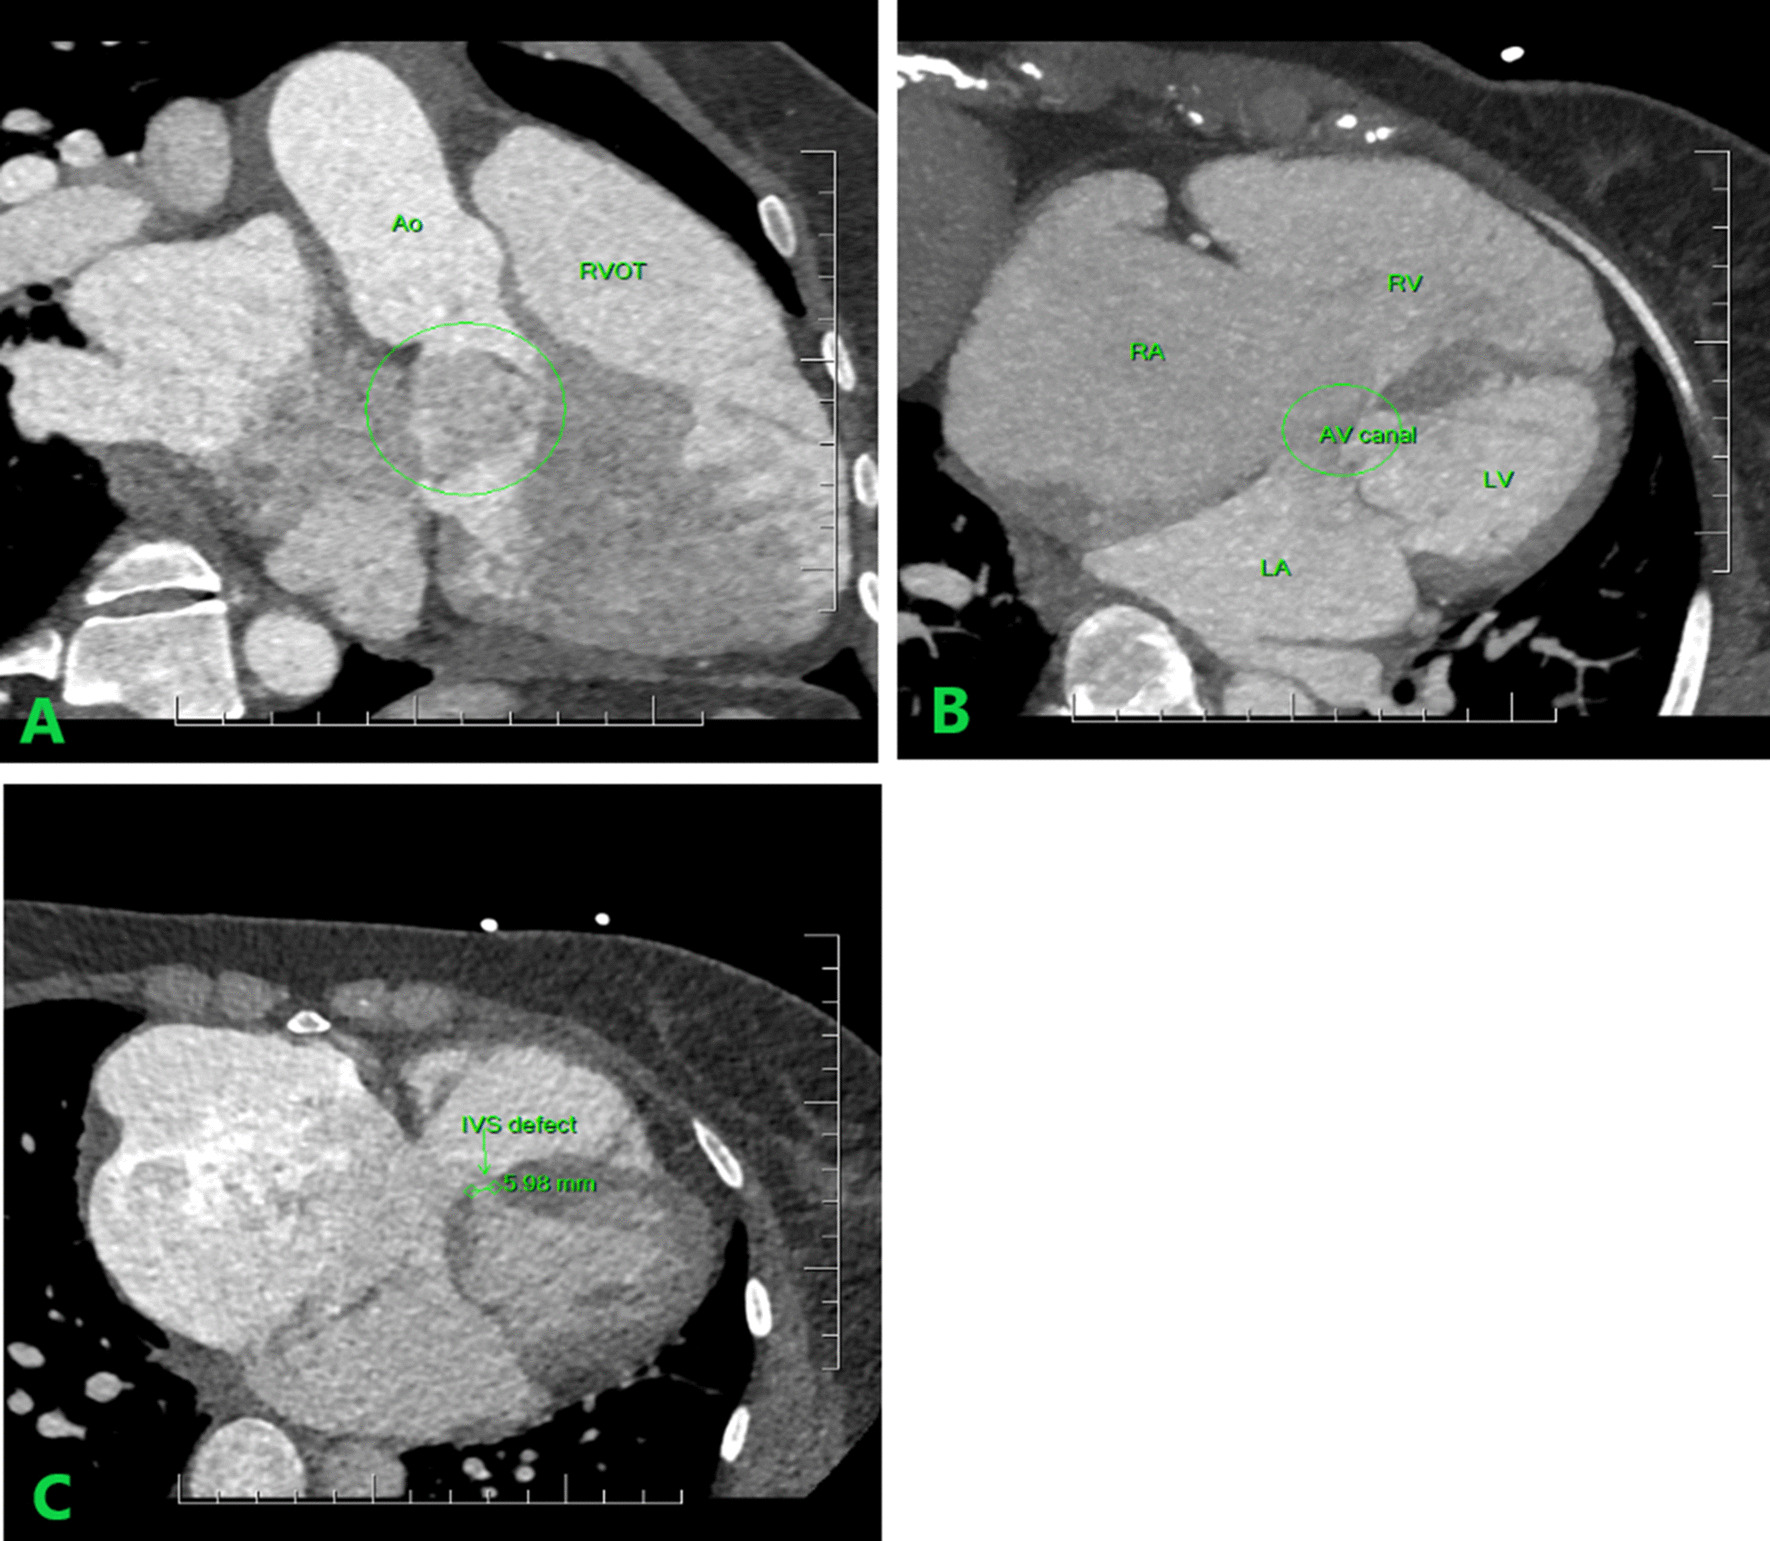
A Atrioventricular septal defect. Four-chamber CCT image showing the open atrioventricular canal valve, B four-chamber CCT image showing the atrioventricular canal, C four-chamber CCT image showing the intraventricular septal defect of 6 mm. *CCT* cardiac computed tomography
